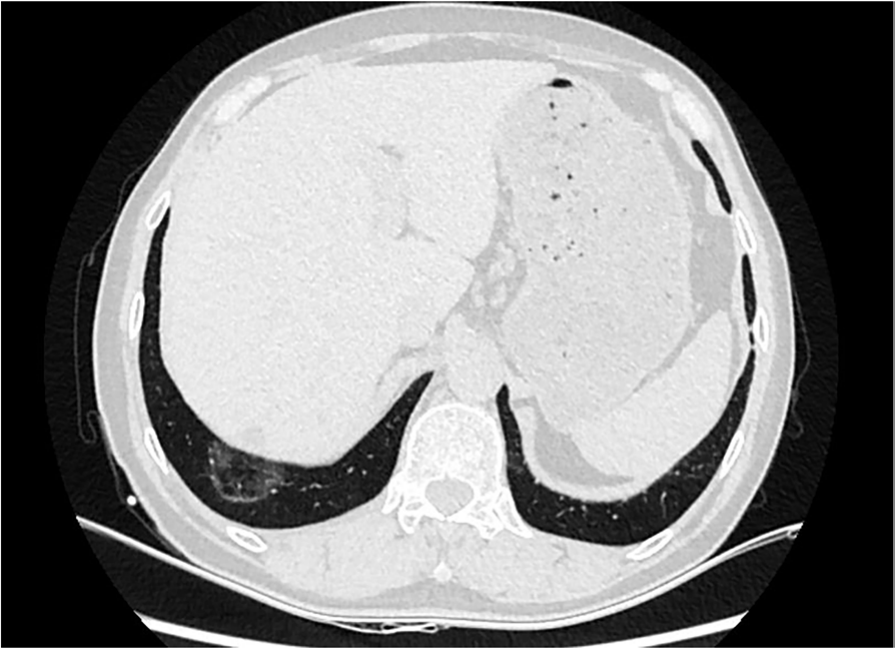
Computed tomography showed ground-glass lesions in the lower lobe of the right lung
